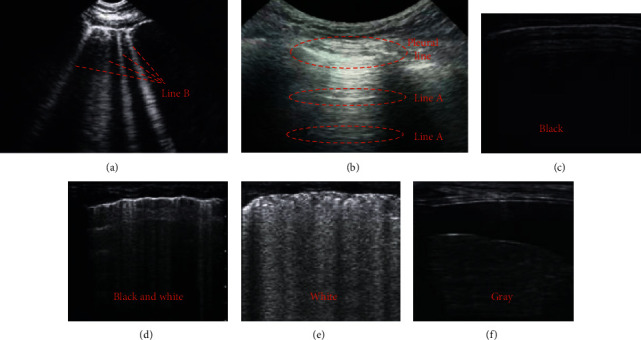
Ultrasound signs of the lungs. (a) A line; (b) B line; (c) normal; (d) mild-to-moderate interstitial edema; (e) severe interstitial edema and alveolar edema; (f) lung consolidation.
